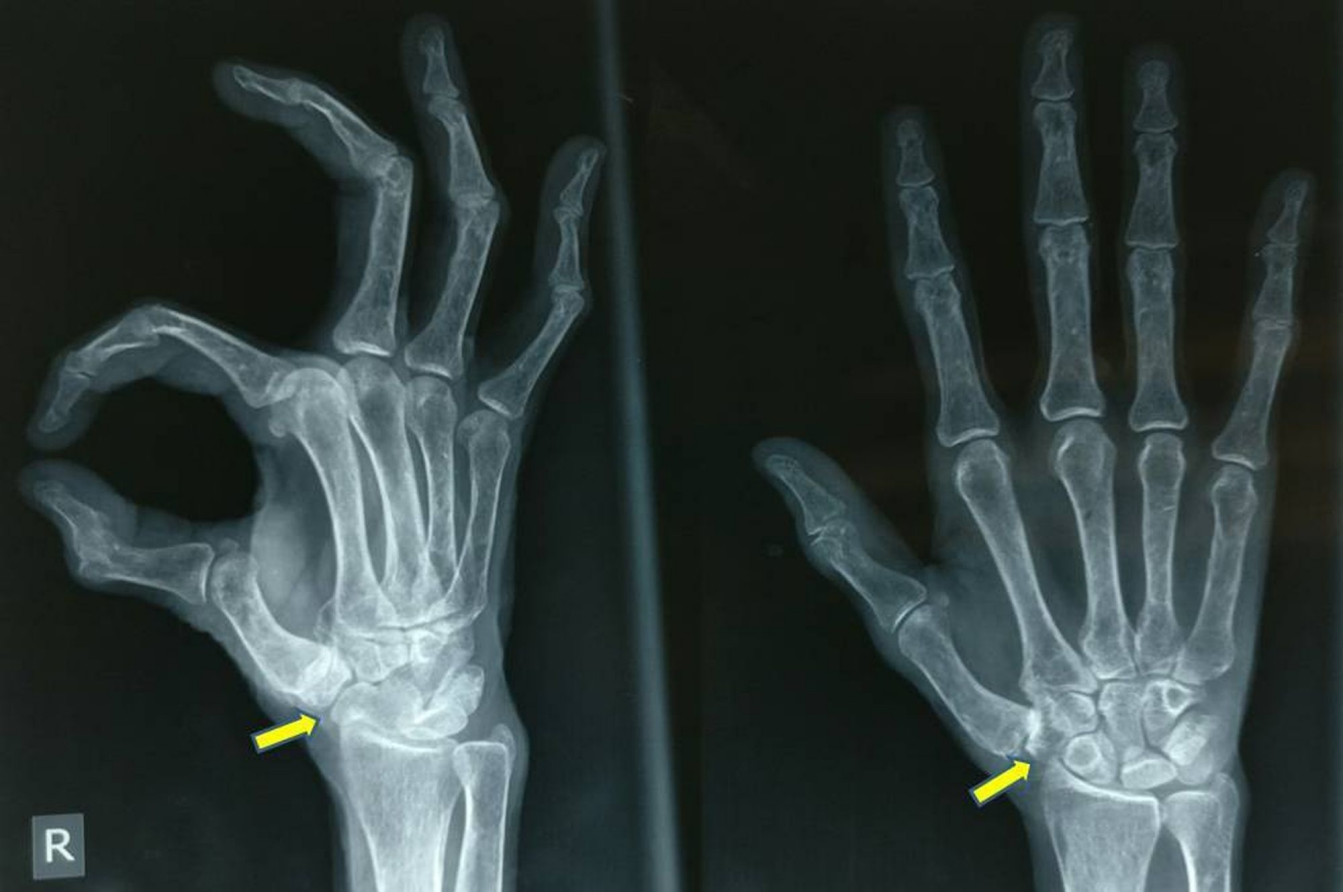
Pre-operative radiograph (antero-posterior and oblique views) Image showing arthritic first metacarpal-trapezium joint.
